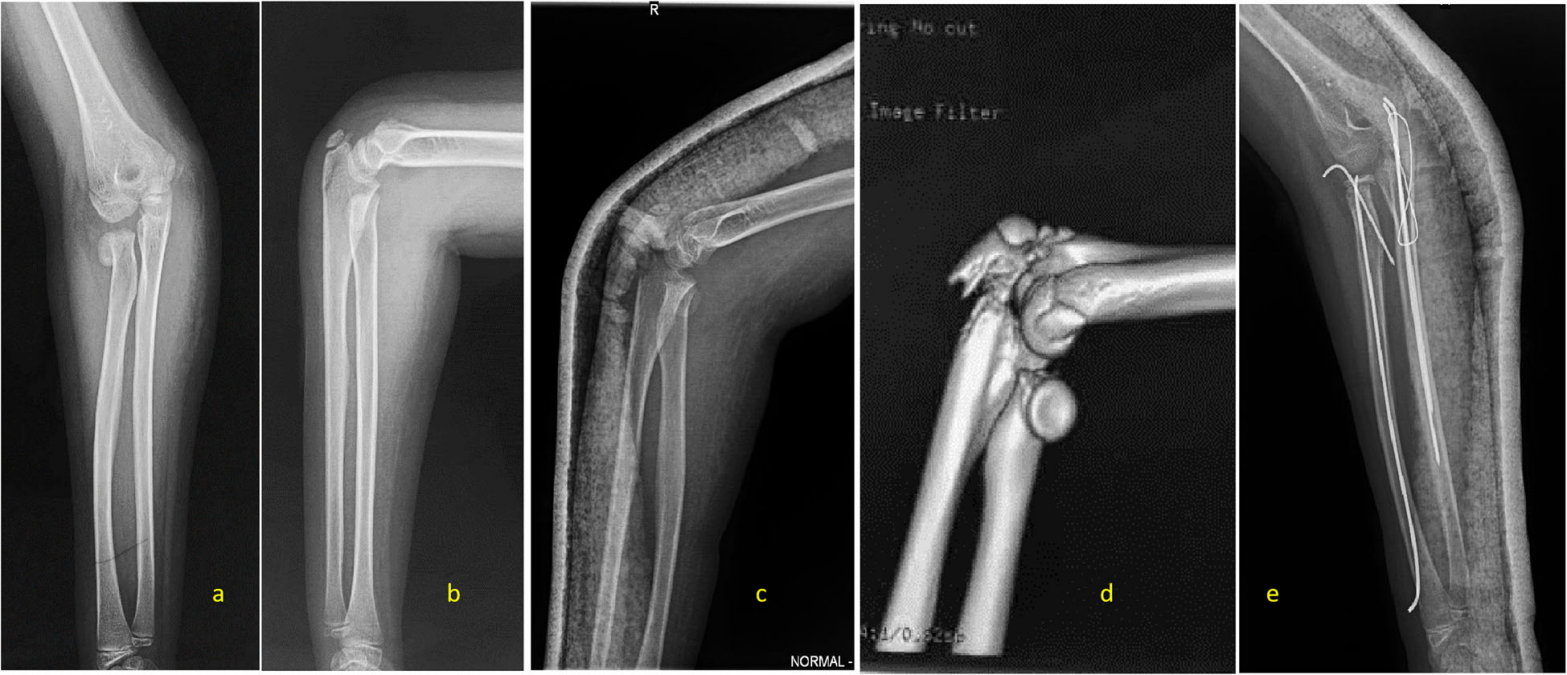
An 8-year-old girl with a type II injury in right elbow. a, b A-P and lateral view of X-ray film show transverse olecranon fracture proximal to the coronoid process without displacement, associated with radial neck fracture with angulation more than 60° or displacement more than two thirds of radial neck. c After 1 week plaster immobilization of the elbow, lateral view of X-ray film shows displacement of olecranon fracture, and a type III 2D fracture. d 3D reconstructed image of CT scan shows the precise fracture configuration. e After open reduction and internal fixation with K-wires for the olecranon and close reduction and internal fixation with a flexible nail and cross pinning for the radial neck
